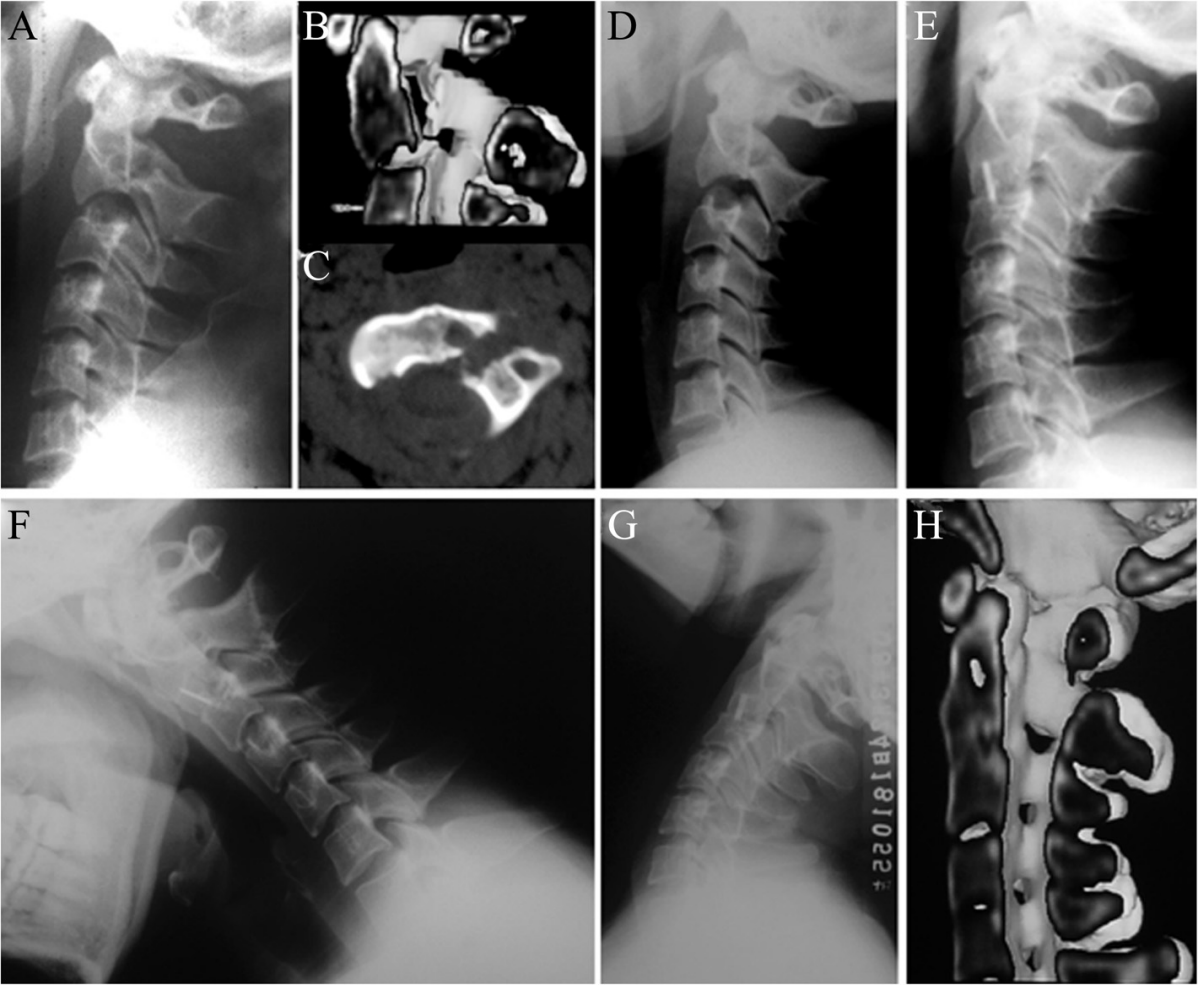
Images of a 38-year-old male patient. a and b, preoperative lateral X-ray and CT scans showing a type IIA hangman’s fracture with severe angulation. c, CT with axial section showing a bone cyst in the vertebral body of C2. d, Some degree of reducing was accomplished during skull traction for 3 days. e, 3-month postoperative lateral X-ray showing adequate reduction and bony fusion. f and g, 24-month flexion/extension lateral X-rays showing no range of motion at the fusion site. h, CT with sagittal reconstruction showing solid fusion and fracture healing
